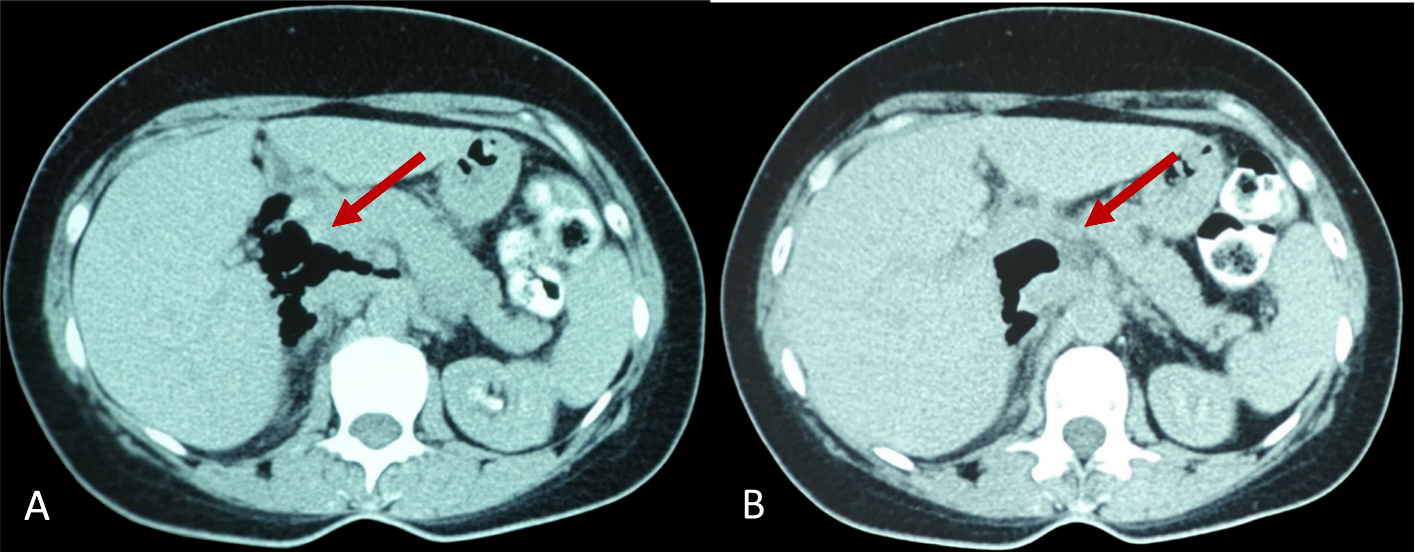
a-b: CT scan of the abdomen, showing free locules of air in the hepatic hilum and the lesser omental sac
